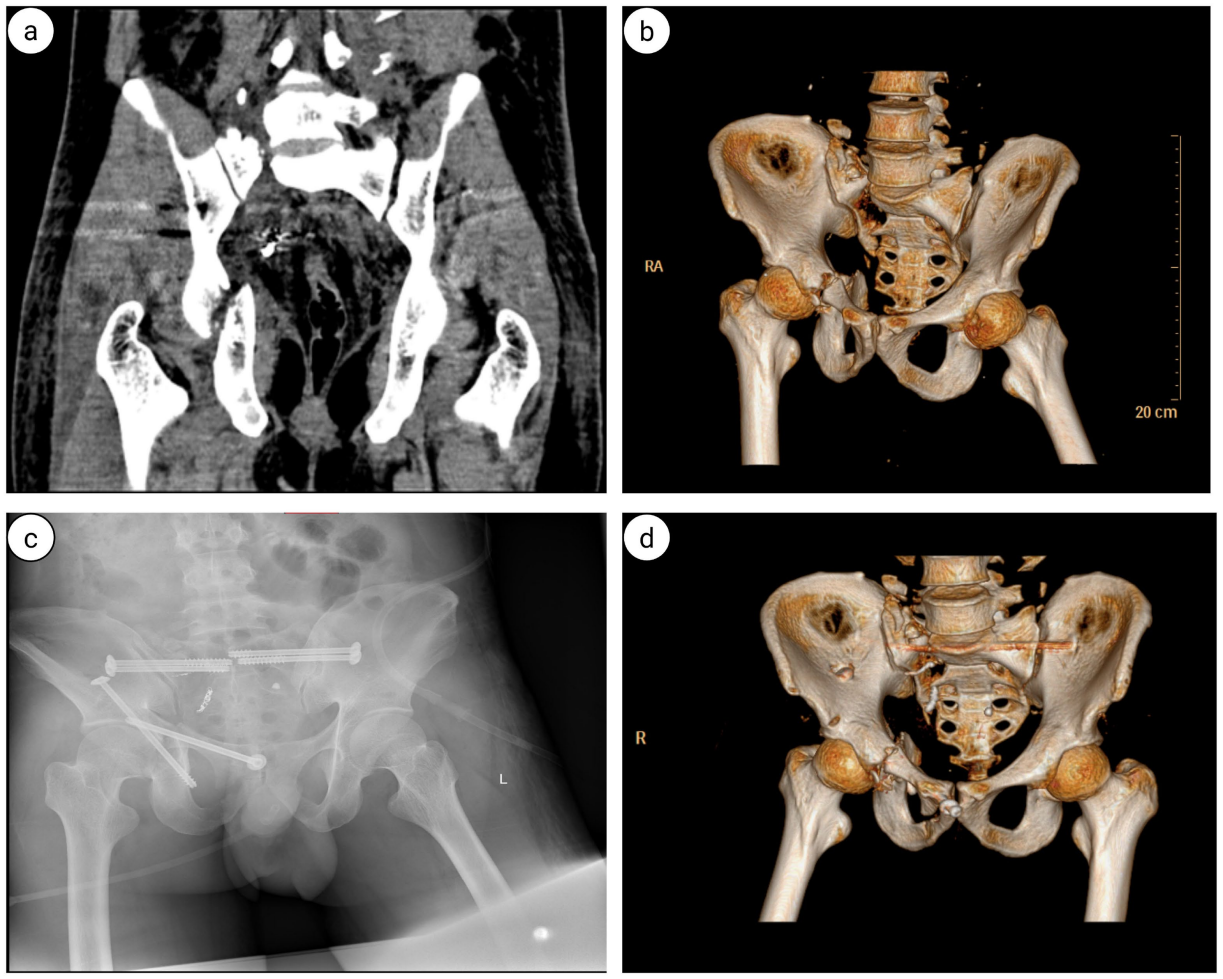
Preoperative and postoperative digital radiography (DR) and three-dimensional computed tomography (3D CT) of pelvic and acetabular fracture: (a) Preoperative pelvic anteroposterior X-ray; (b) preoperative pelvic computed tomography (CT) three-dimensional reconstruction; (c) postoperative day one pelvic anteroposterior X-ray; (d) postoperative day one pelvic CT three-dimensional reconstruction.
